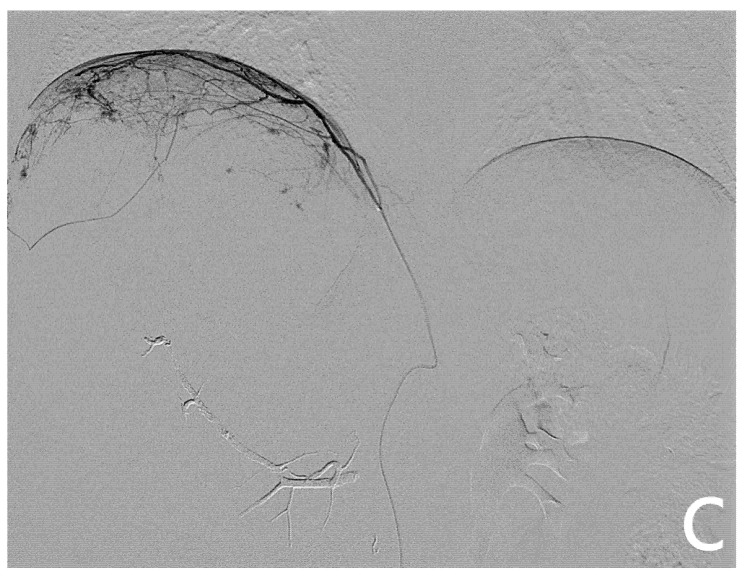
On preoperative CT (a), a giant hemangioma of the right liver lobe is identified, with evidence of vascular afference from the right phrenic artery (arrow). On angiography, after identification and embolization of the right hepatic artery (b), right phrenic artery is selectively catheterized and embolized with microparticles (c).
